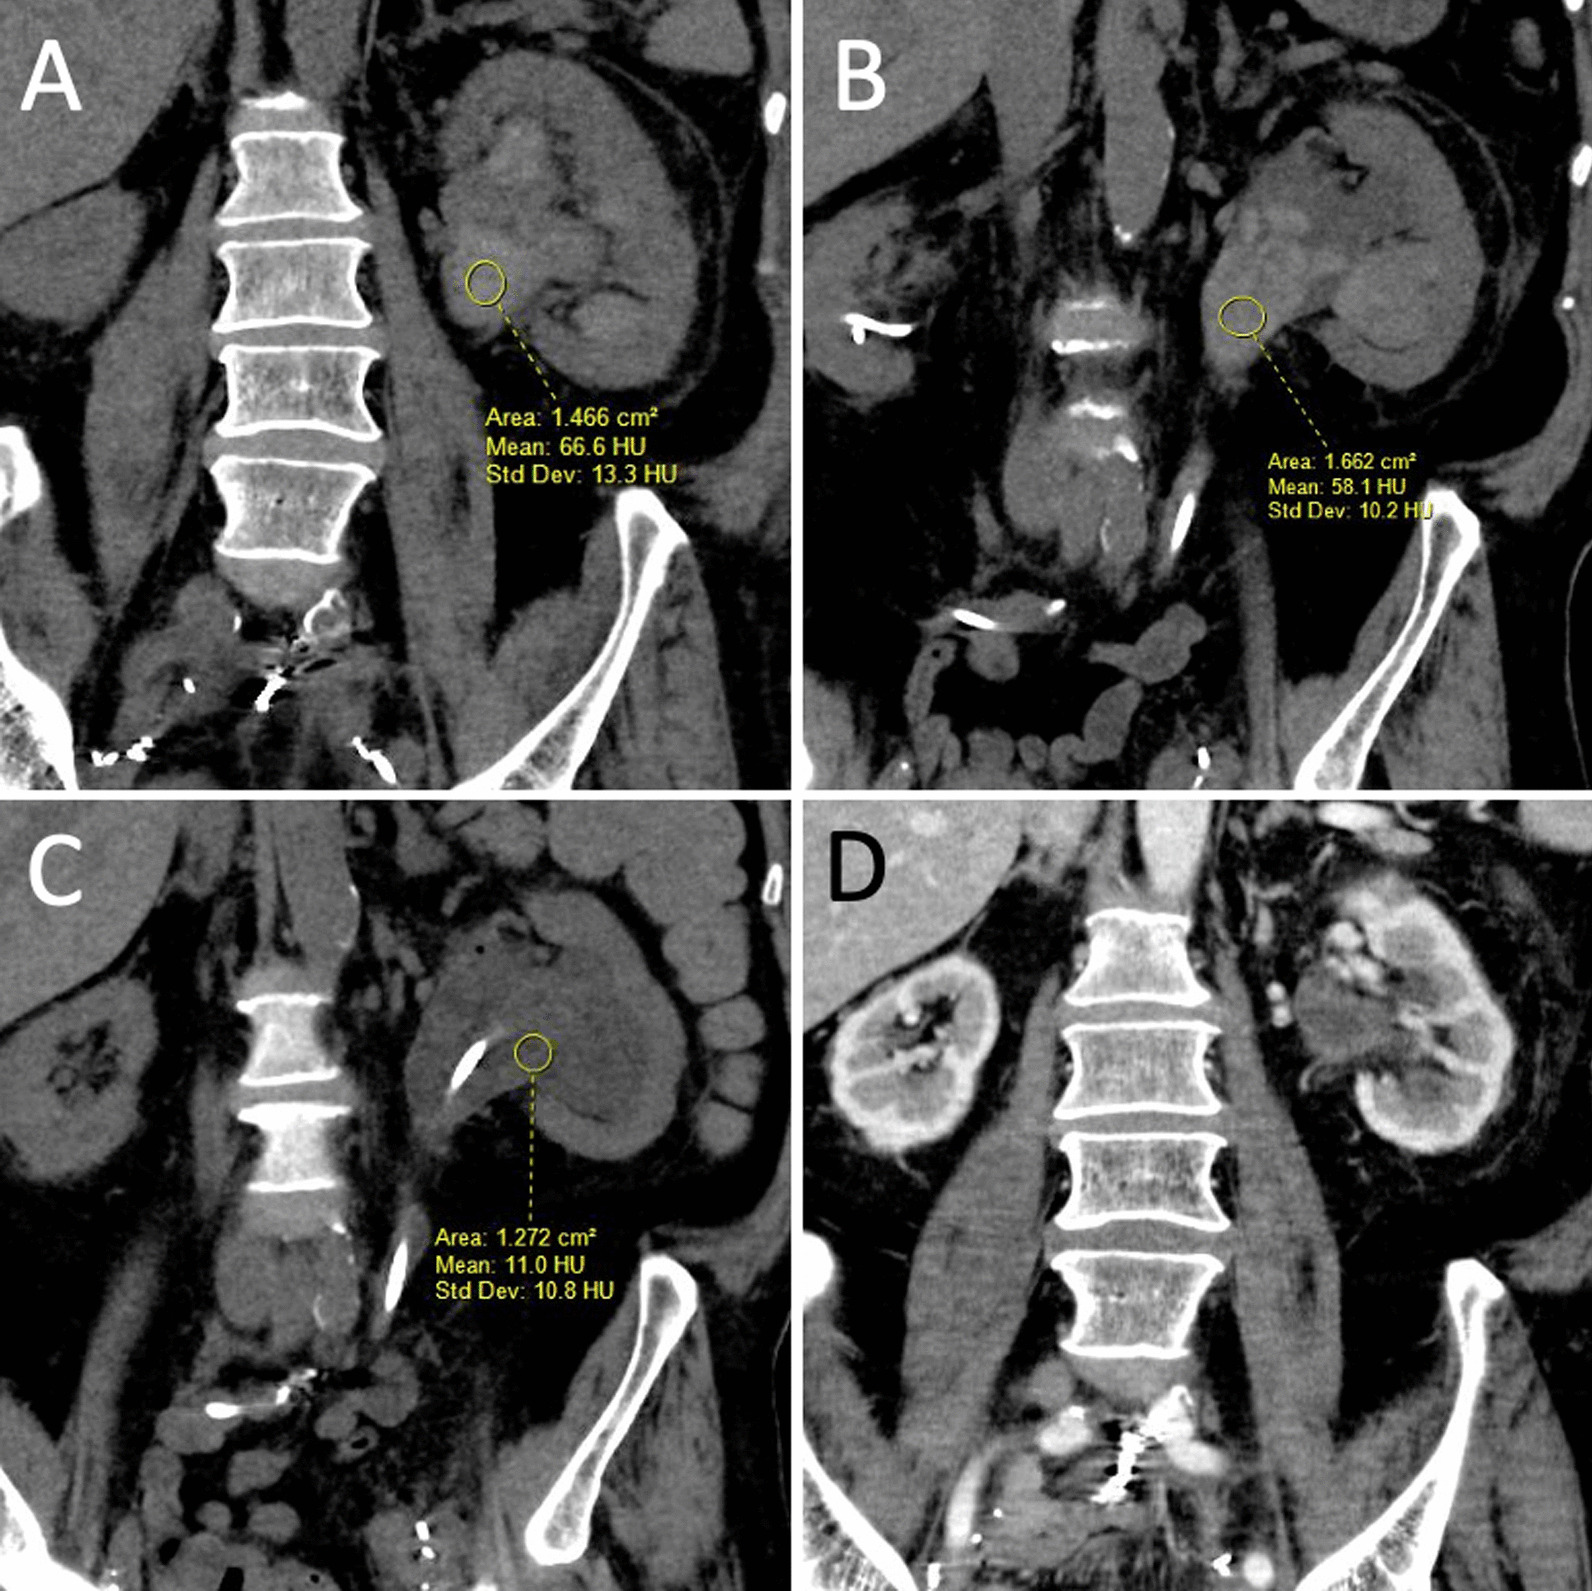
CT of the abdomen and pelvis at different time points, demonstrate large blood clots in the left pelviureteric system with significant left hydronephrosis not significantly different between two consecutive CT scans, A from 11 days prior to- and B from two days post- embolization. C Interval resolution of pelviuretric dense blood clots 17 days post embolization, but persistent significant hydronephrosis. D Interval resolution of hydronephrosis 45 days post embolization; no evidence of recurrence of dense blood clots
